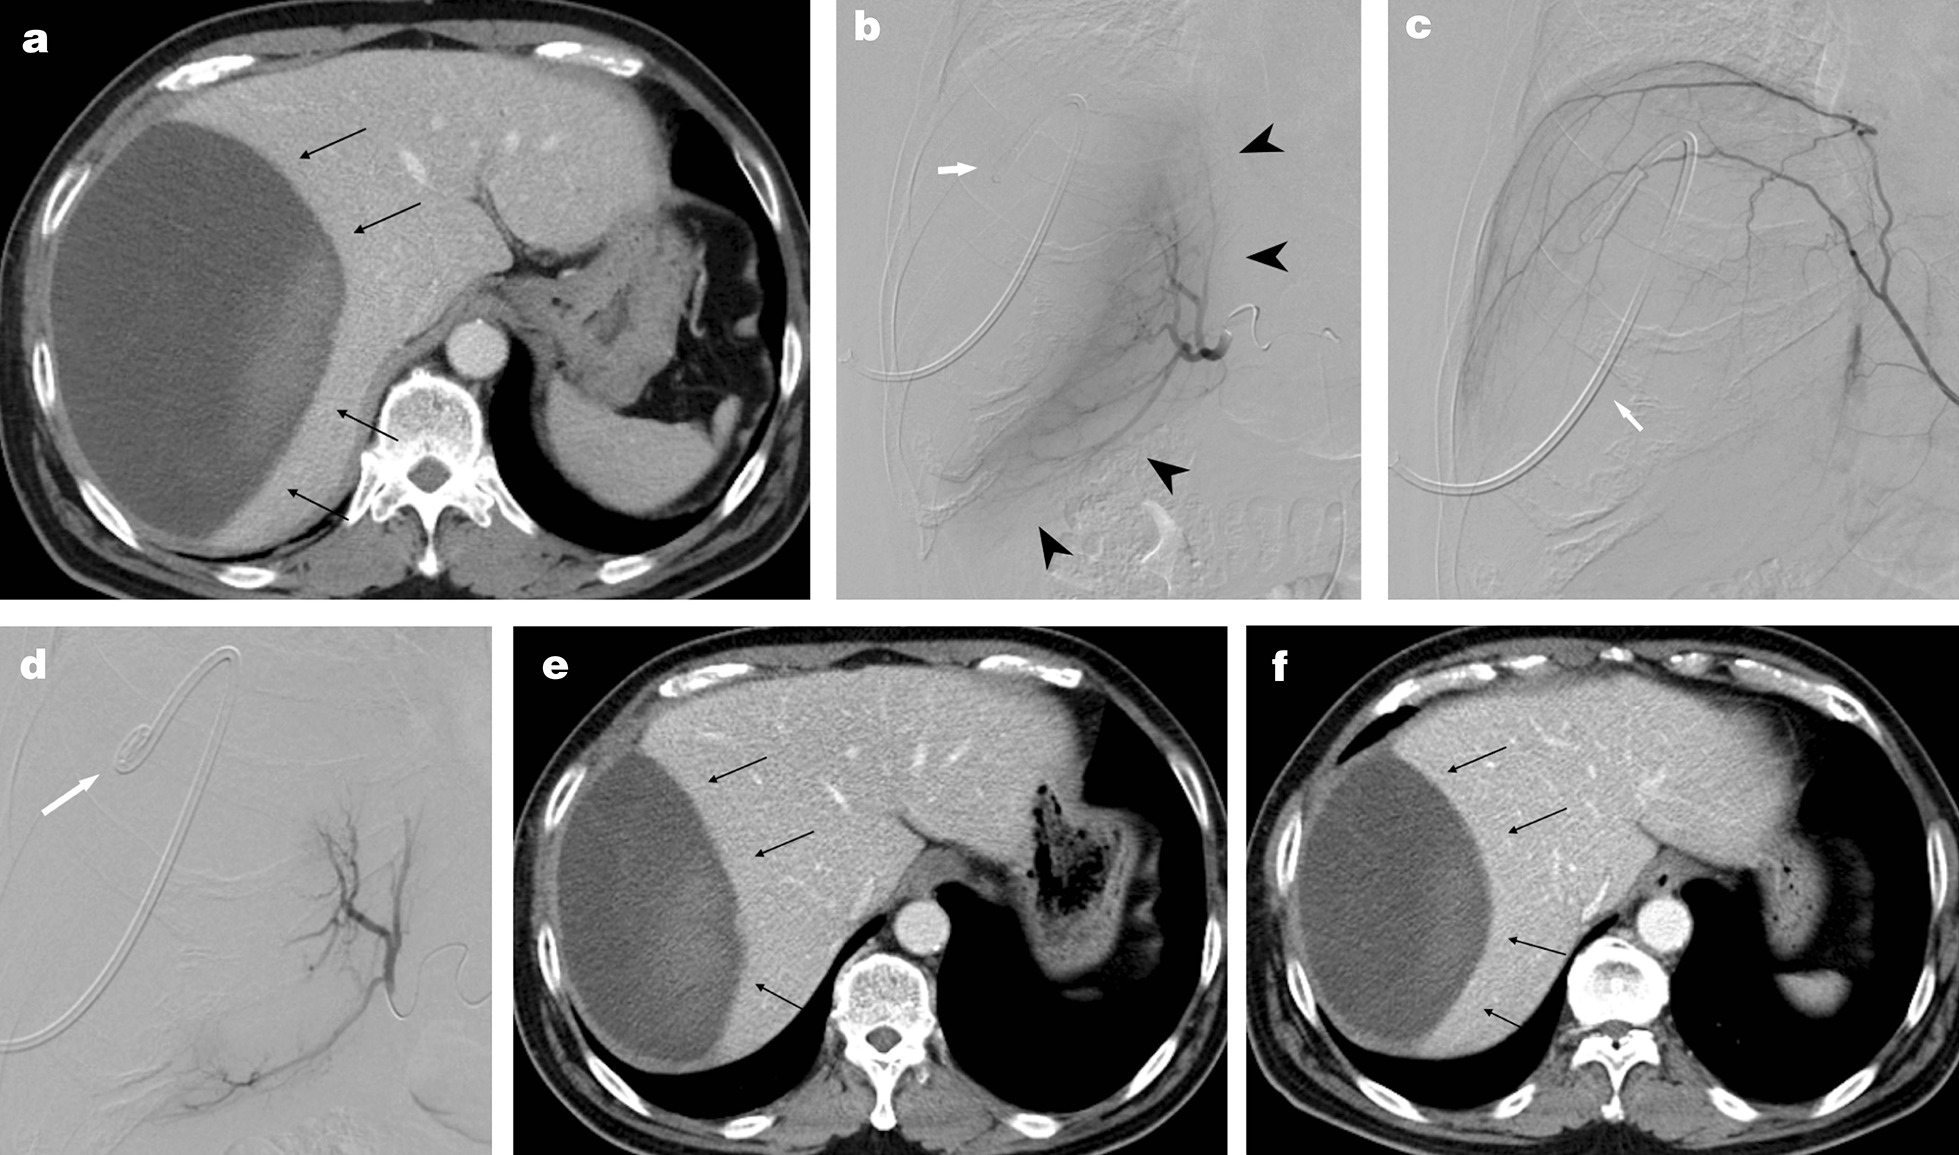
A 56-year-old man with a subcapsular hepatic hematoma diagnosed by abdominal computed tomography (CT). a Contrast-enhanced abdominal CT at the time of diagnosis. The hematoma is visible beneath the capsule of the right hepatic lobe (black arrows). b–d Percutaneous CT-guided drainage and transarterial embolization (TAE) are performed on Day 34 after the initial examination. b Digital subtraction angiography of the right hepatic artery. This artery is deviated, and the hepatic parenchyma is displaced by the hematoma (black arrowheads). The white arrow indicates the drainage catheter. There is no sign of contrast medium extravasation or an isolated artery. c Digital subtraction angiography of the right inferior phrenic artery. There is no sign of contrast medium extravasation or an isolated artery. d Digital subtraction angiography of the right hepatic artery after embolization. Blood flow is stopped in the periphery of the right hepatic artery. e Contrast-enhanced abdominal CT after percutaneous CT-guided drainage and TAE. The hematoma (black arrows) can be seen to have decreased in size. f Contrast-enhanced abdominal CT 1 month after TAE. The hematoma (black arrows) has not been resorbed, but it has started to regrow
